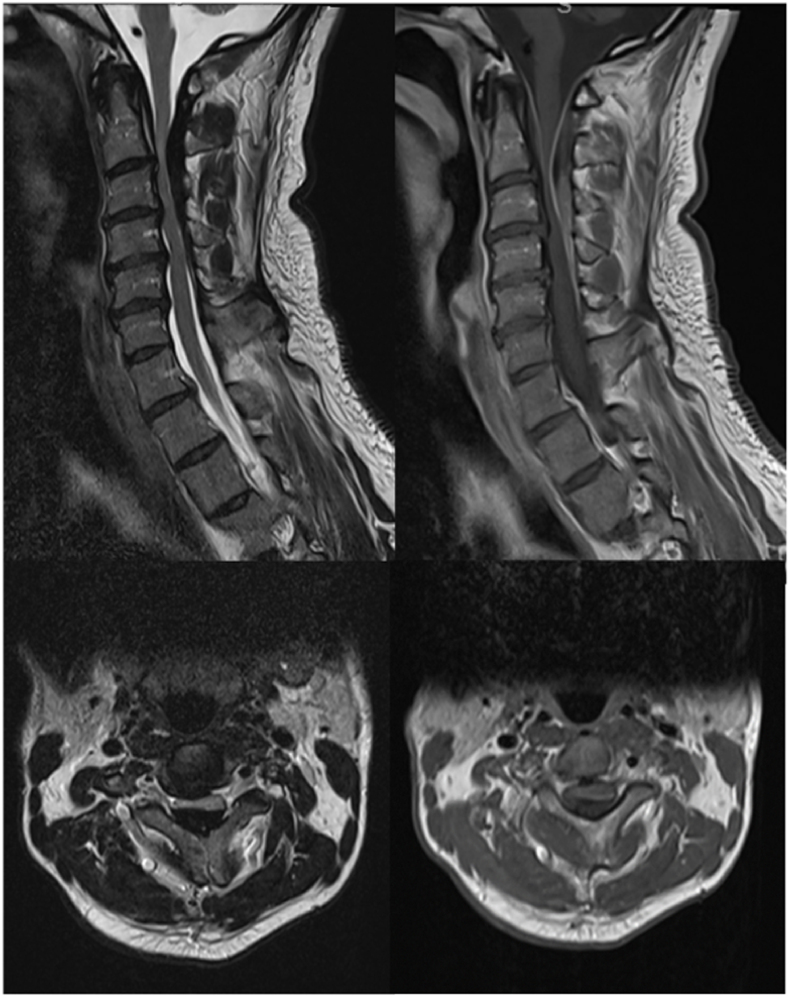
MR-imaging (T2-weighted on the left, T1 with gadolinium on the right) demonstrating thickening of the ventral and dorsal meninges from C2 up to C5 resulting in absolute spinal canal stenosis. Additional post-Gadolinium T1-weighted images show a strong enhancement of the dura mater.
